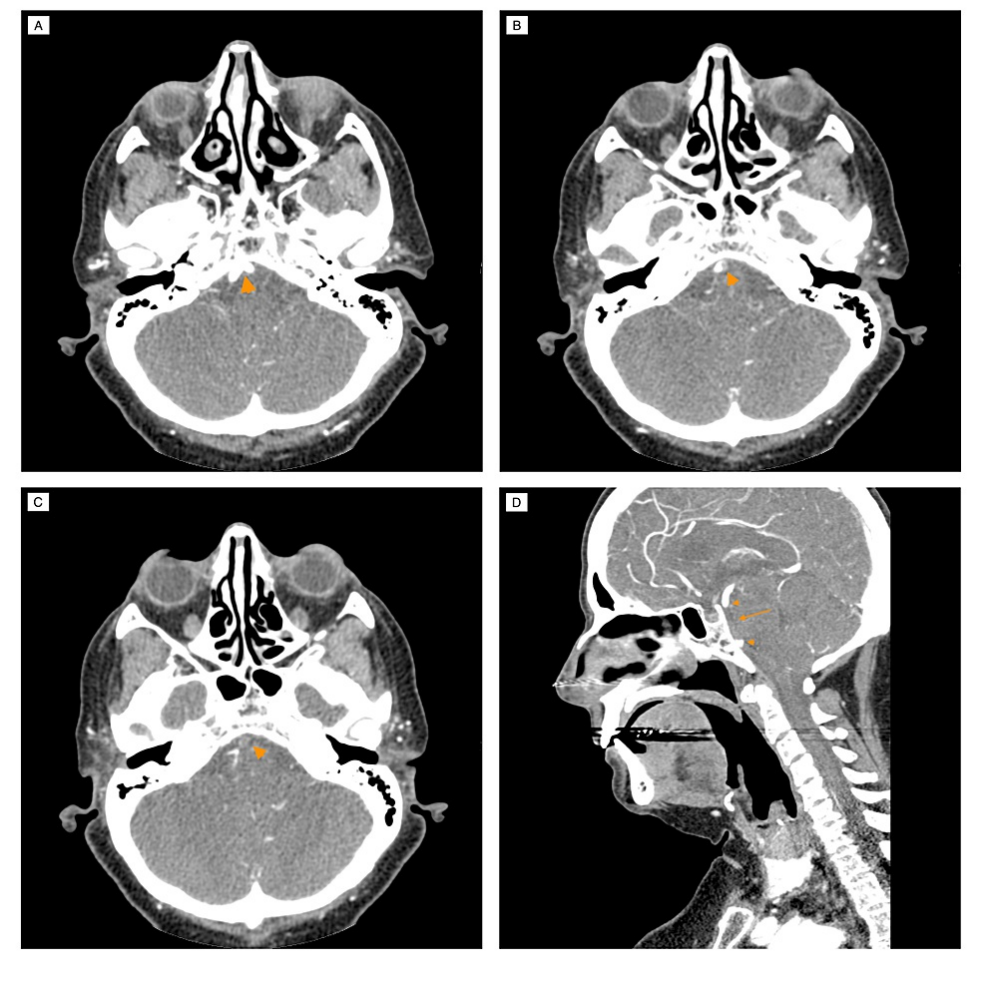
Computed tomography cerebral angiography showing basilar artery occlusion. (A) shows both vertebral arteries joining to form the basilar artery (arrowhead). (B) shows the beginning of the basilar artery with a filling defect caused by an endoluminal thrombus (arrowhead). (C) shows total absence of contrast opacification of the basilar artery (arrowhead). (D) is a reconstructed sagittal section showing the extent of the basilar artery thrombus, occupying its proximal and middle segments (arrow); contrast opacification stops at the beginning of the basilar artery (arrowhead) and is again evident at the distal portion of the basilar artery (arrowhead) because of retrograde flow through the anterior circulation.
